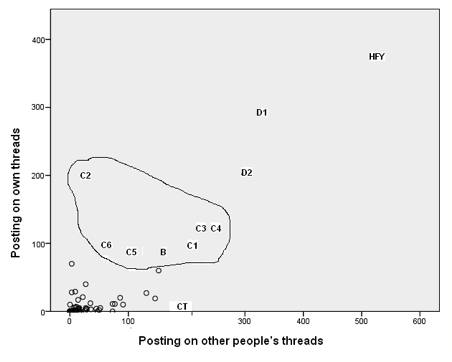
Number of postings on their own threads versus postings on other people’s threads showing Here for you (HFY), Discussants 1 and 2 (D1 and D2), Butterfly (B), Caretaker (CT), and Crisis-oriented 1-6 (C1 to C6)
